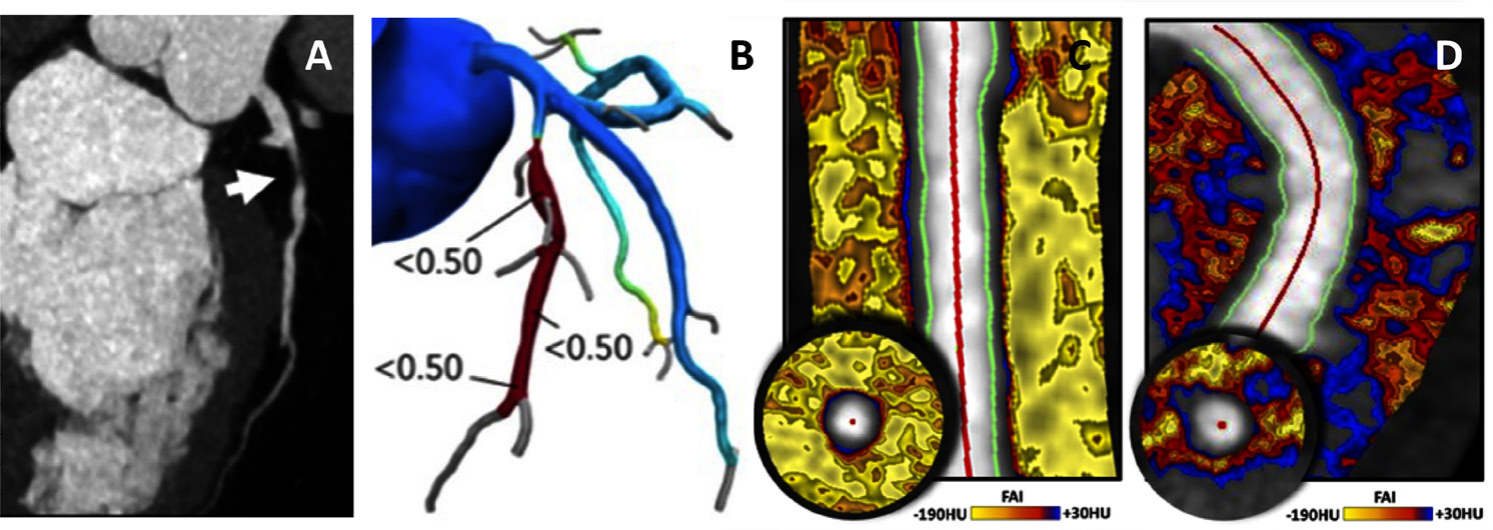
CAD characteristics evaluated by CCTA. (A) Non-calcified, causing a significant luminal narrowing (70%–99%), with (B) computational CT-fractional flow reserve (FFR) indicating a significant FFR across the lesion. (C) Low Fat Attenuation Index (FAI) of the perivascular adipose tissue (predominant yellow shading), whereas an angiographically similar coronary segment shows marked areas of low-attenuation perivascular adipose tissue (red-blue), indicating inflammation and a high risk of future cardiovascular events, despite no apparent luminal atheroma. CAD, coronary artery disease; CCTA, coronary CT angiography.
